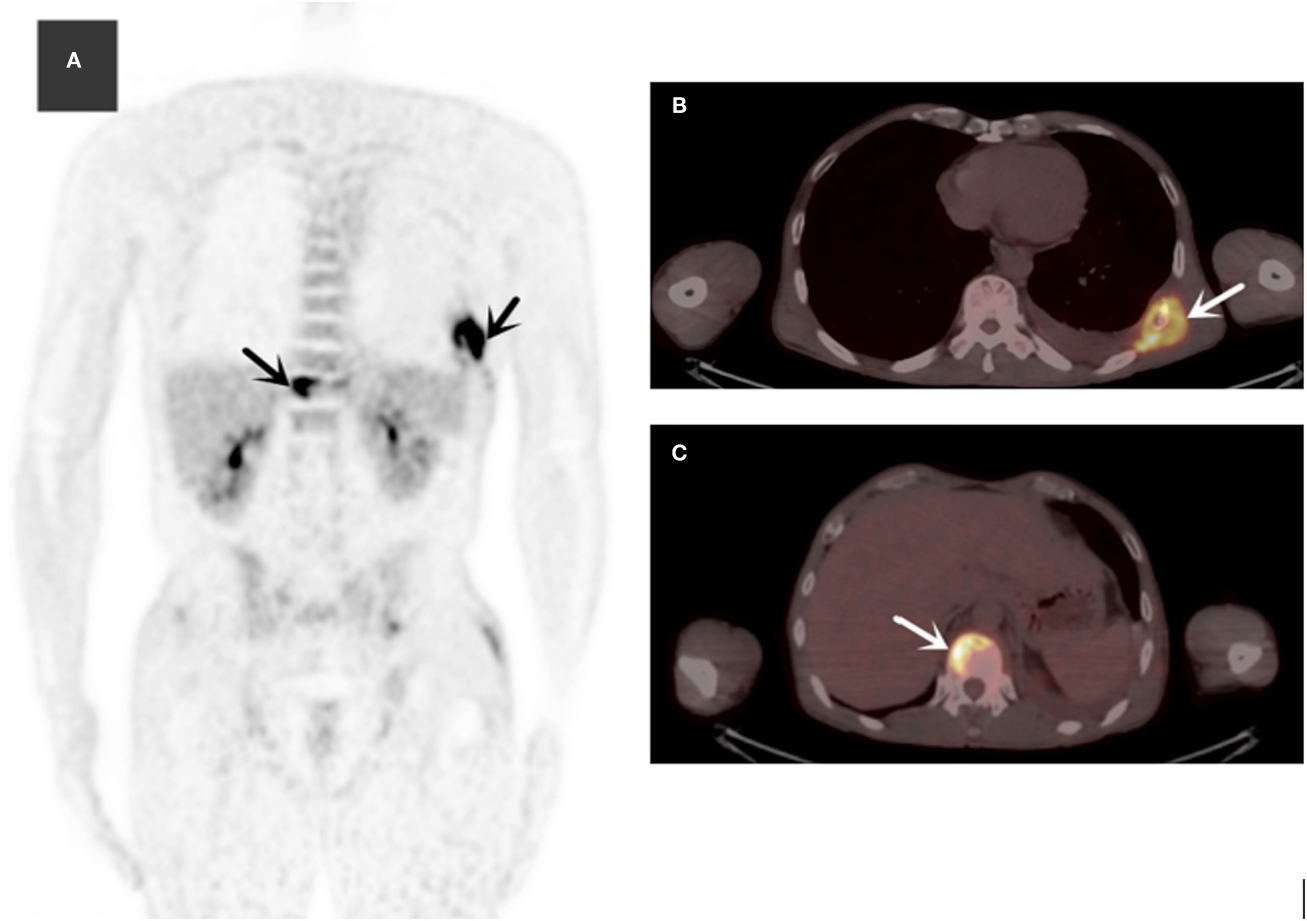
The MIP (maximum intensity projection) of 18F-FDG (fluoro-D-glucose) PET/CT (A) revealed multiple hypermetabolic lesions in the whole body (black arrows). Axial slices showed normal ribs (B) (SUVmax = 10.3) and multiple hypermetabolic lesions of the spine (C) (white arrows).
